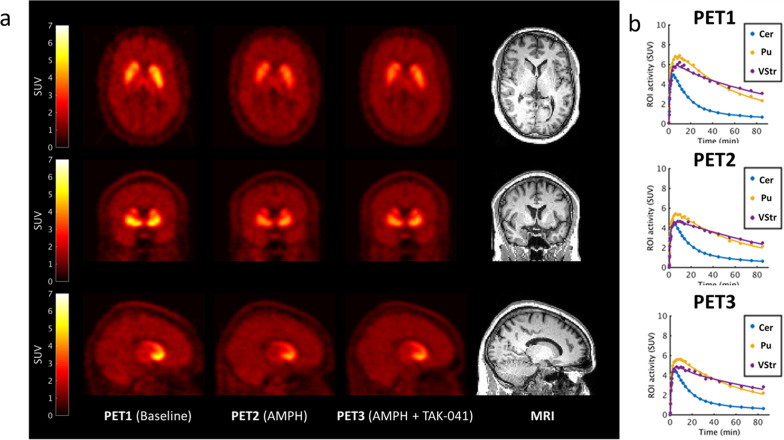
Brain images and time activity curves. a Orthogonal cross-sections of co-registered PET and MR images from a representative participant who received TAK-041 40 mg. For columns from left to right, images are PET1 (baseline), PET2 (post d-AMPH), PET3 (post d-AMPH + TAK-041), and structural (T1-weighted) MRI. PET images are shown as SUV summed from 10 to 90 min; b Time–activity curves and simplified reference tissue model fits (using cerebellum as reference region) for a representative participant (participant 10). AMPH amphetamine, Cer cerebellum, MRI magnetic resonance imaging, PET positron emission tomography, SUV standard uptake value, Pu putamen, ROI region of interest, VSt ventral striatum.
